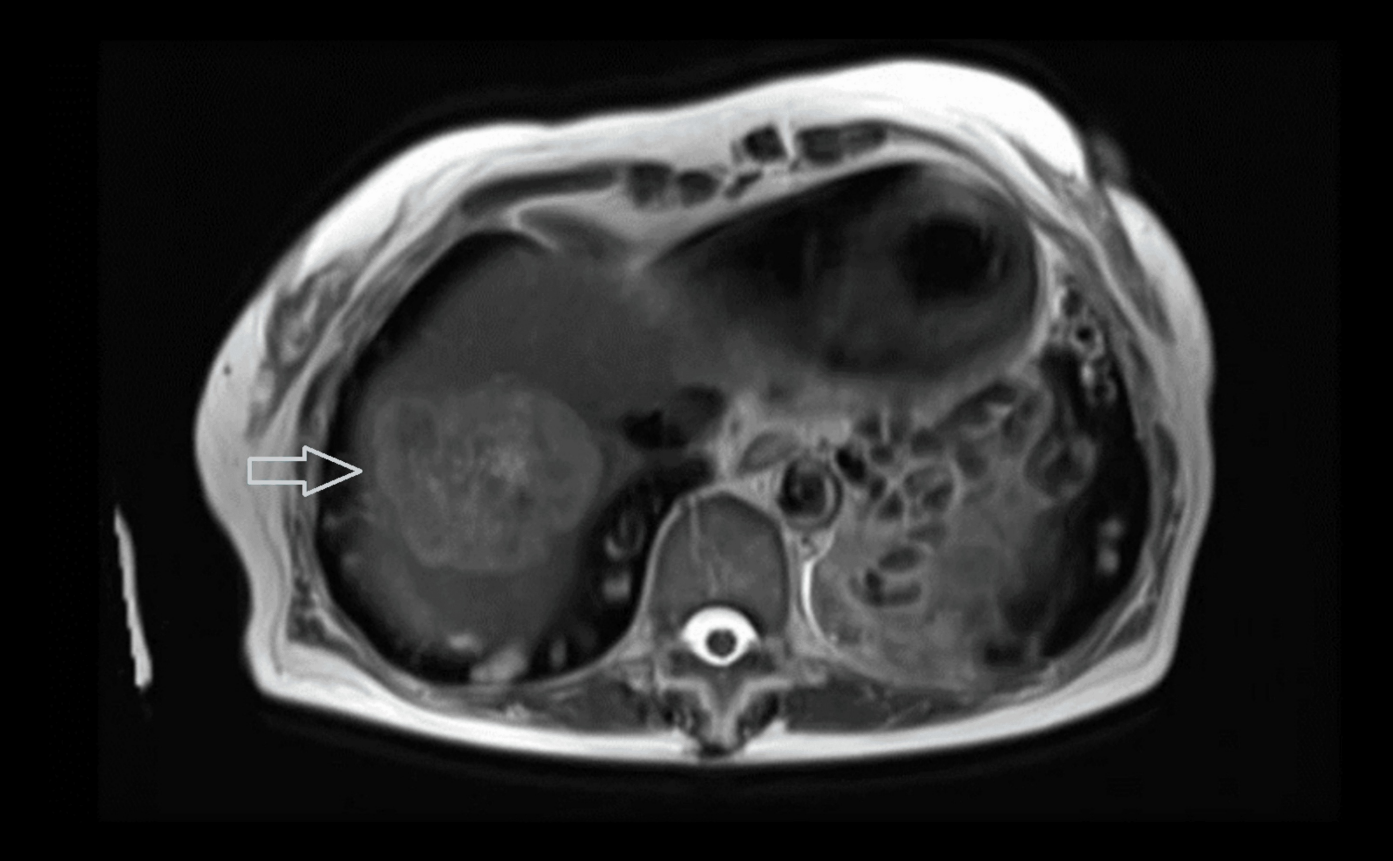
Axial view of MRI liver showing a large 5.2 x4.6 cm mass in the right lobe of liver with patchy high-signal intensity change seen on T2 weighted images
